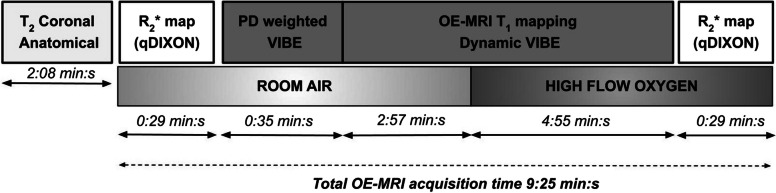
Study sequences and timing of switch from room air to high flow oxygen. Sequence parameters provided in Table 1. *OE-MRI* Oxygen-enhanced magnetic resonance imaging, *VIBE* Volumetric interpolated breath-hold examination
